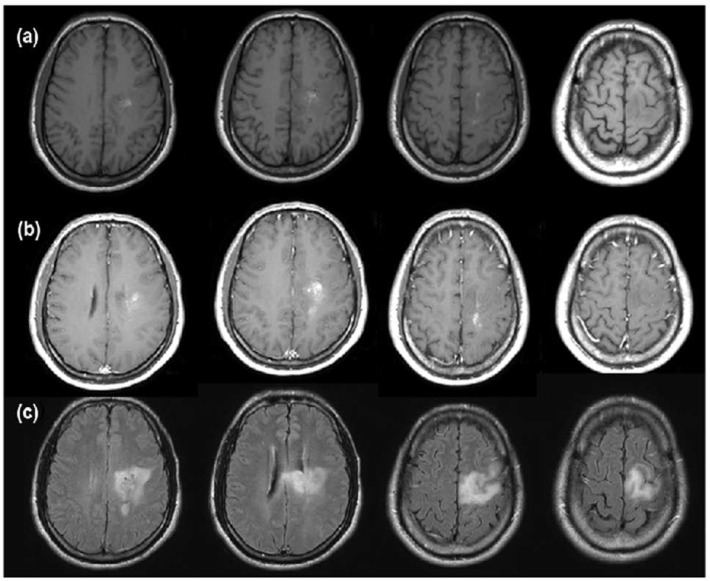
Brain magnetic resonance imaging (MRI) findings, (a) Axial T1-weighted; (b) axial, gadolinium-enhanced, T1-weighted; (c) axial fluid-attenuated inversion recovery. Brain MRI showed an enhancing infiltrating mass lesion in the left paracentral area (a, b), focal high signal is also noted on T1-weighted images, suggesting hemorrhage in the mass (a), MRI findings suggest malignant glial tumor such as malignant astrocytoma or malignant oligodendroglioma.
